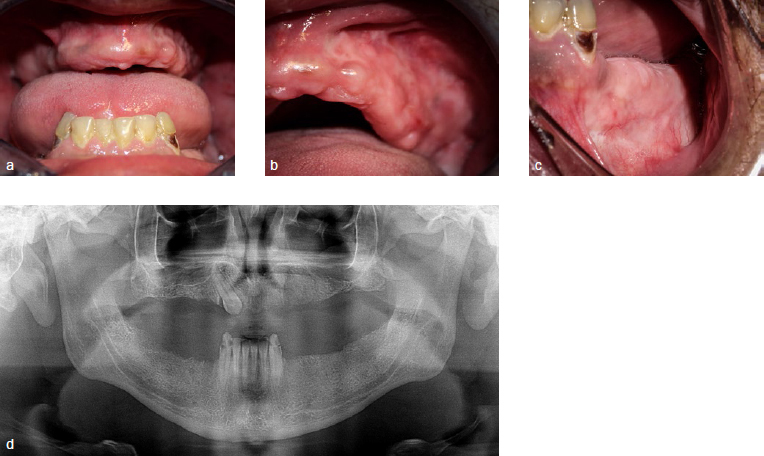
a) Follow up of the oral lesions one year after diagnosis. Patient is stable and pending prosthetic dental rehabilitation. b) Healed ulcer in maxilla. c) Patient scheduled to have conservative treatment in teeth of the mandible. d) Panoramic radiograph (December 2018).
